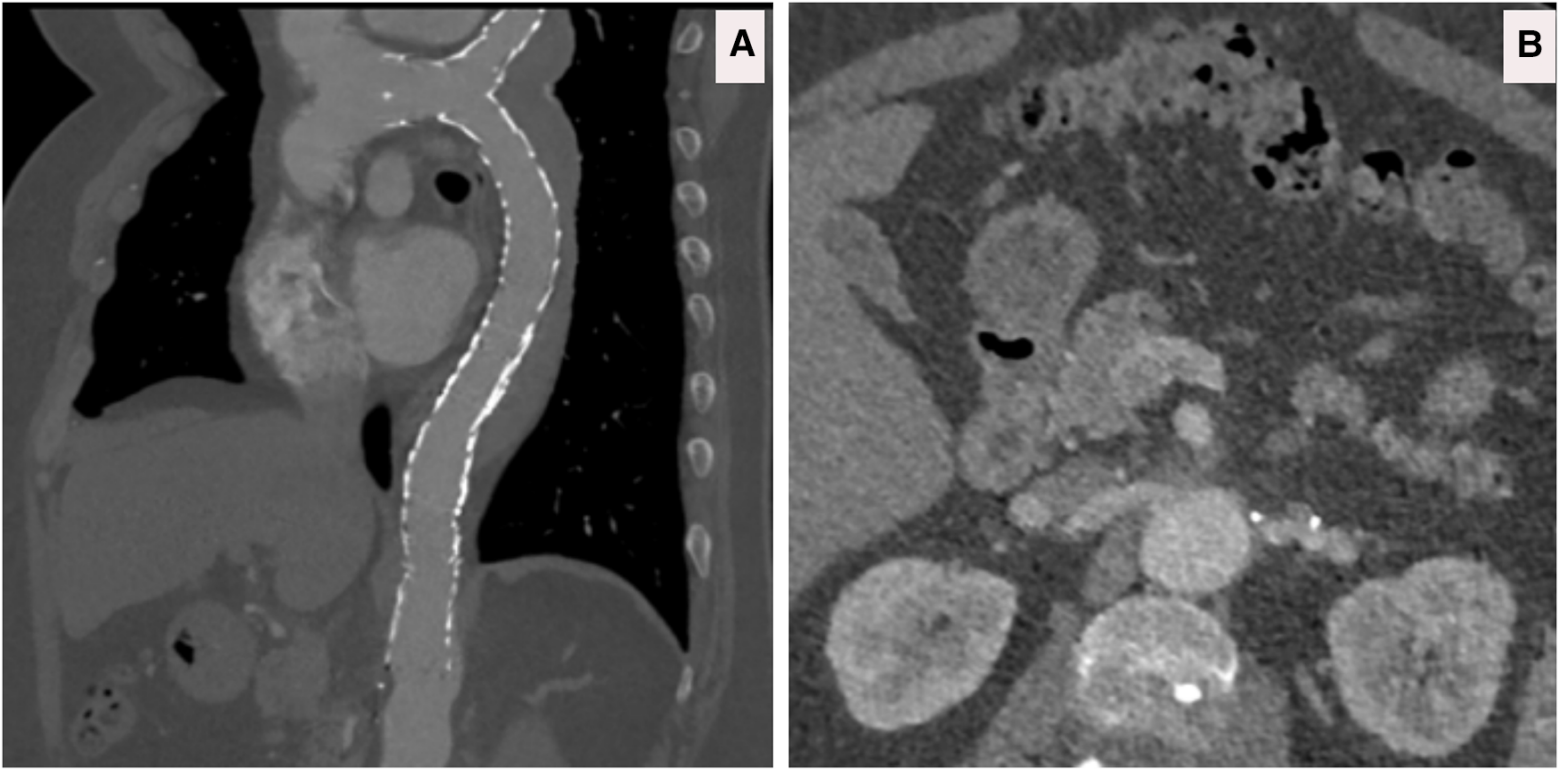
(A) CTA sagittal views confirming the patency of the left subclavian chimney and the thoracic endovascular aortic repair (TEVAR) gore C-TAG graft. (B) CTA axial views, five years post sTaged hybrId sinGle lumEn Reconstruction (TIGER), show well-perfused kidneys without any evidence of dissection, aneurysmal dilatation or restenosis.
